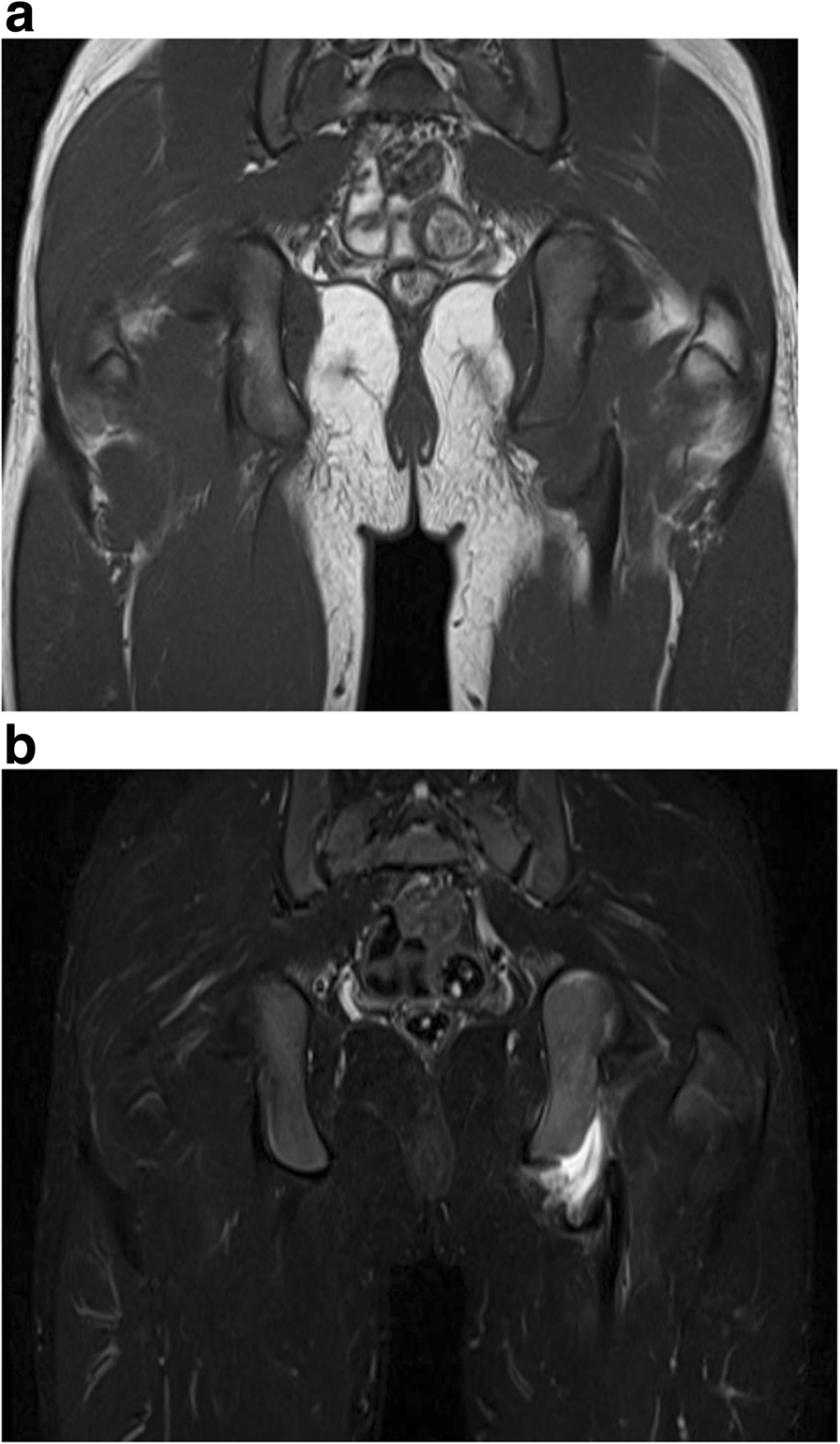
MRI two months postinjury reveals effusion at the area of epiphysiolysis and shows that the muscles have not been directly injured. a T1-weighted MRI. b T2-weighted short TI inversion recovery (STIR)
